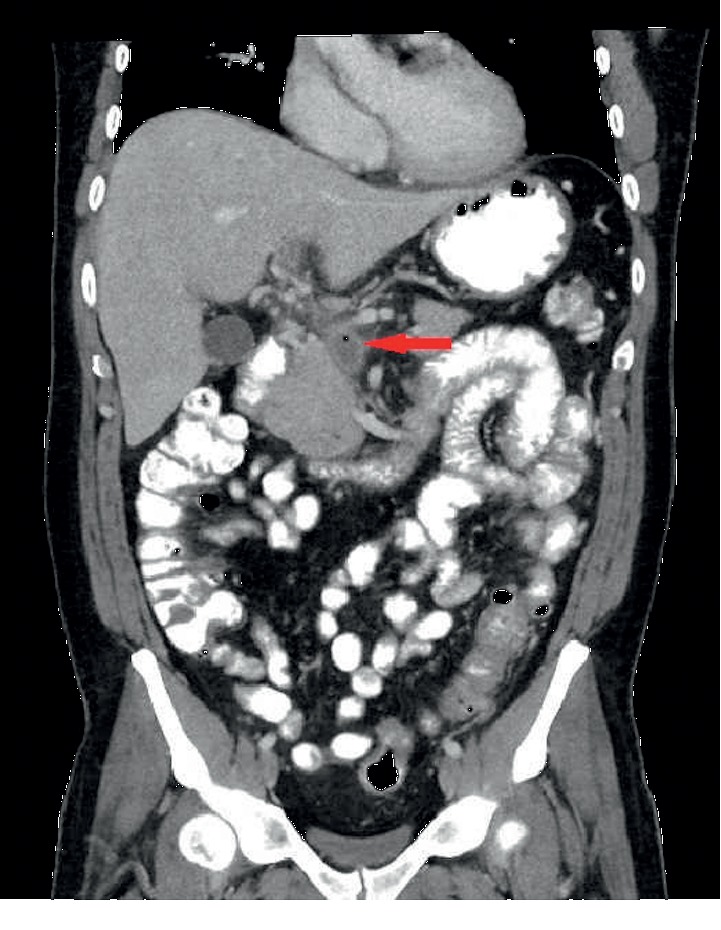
Computed tomography (CT) abdomen was repeated which showed resolution of the portal vein gas but showed interval development of portal vein thrombosis and proximal superior mesenteric vein.
